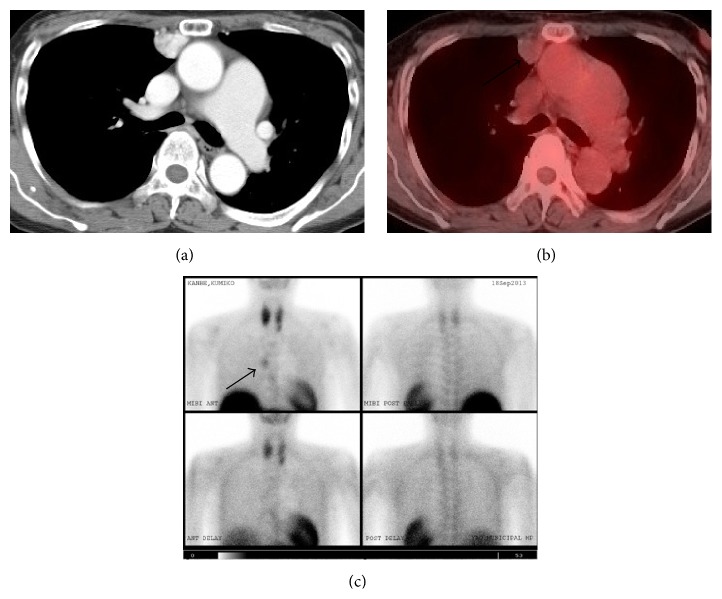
Mediastinal nodule (arrow). (a) CT revealed the presence of a 20 mm nodule in the anterior mediastinum. (b) PET-CT imaging revealed FDG uptake in the nodule. (c) Tc 99m MIBI scan showing an intense focus in the anterior mediastinum.
